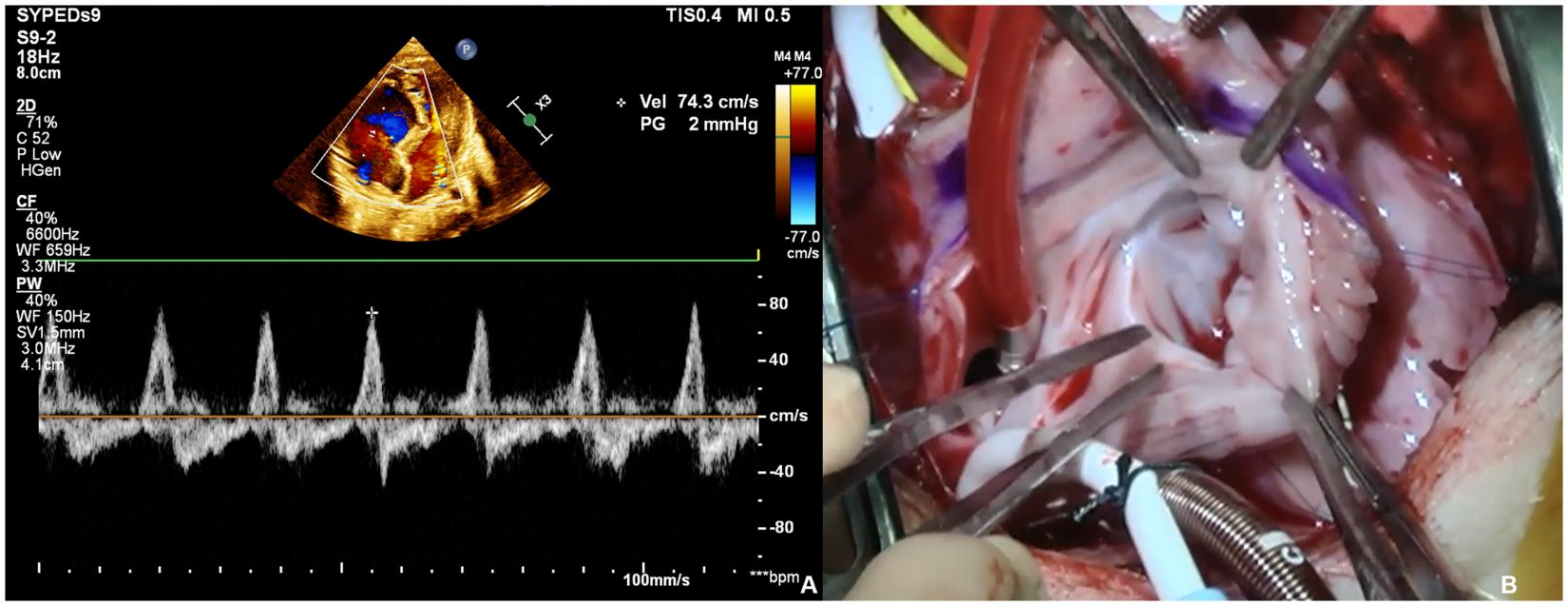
(A) Pulsed Doppler Image of Trans-Thoracic Echocardiogram Showed Free Regurgitation on the Tricuspid Valve Position and (B) Intraoperative Inspections of UGTO.
